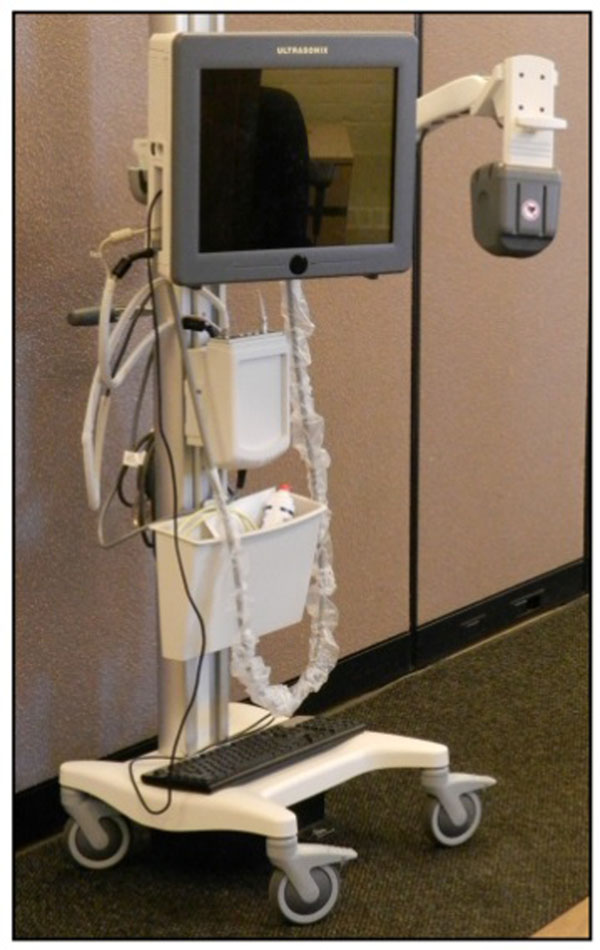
The 3D medical ultrasound machine
